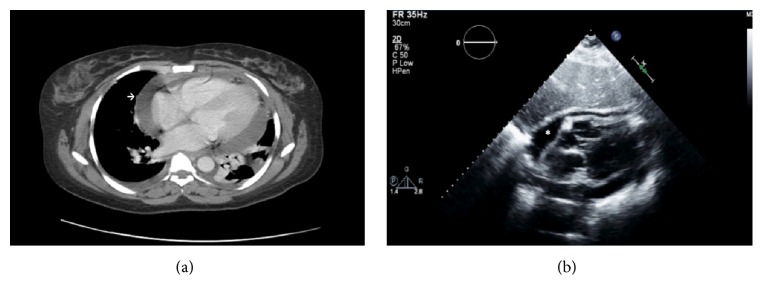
(a) CT showed cardiomegaly with large pericardial effusion (arrow). Bilateral pleural effusions. Left lower lobe is nearly completely collapsed. (b) Parasternal long-axis echocardiogram showed pericardial effusion of moderate size with diastolic compression of the right atrium (asterisk).
